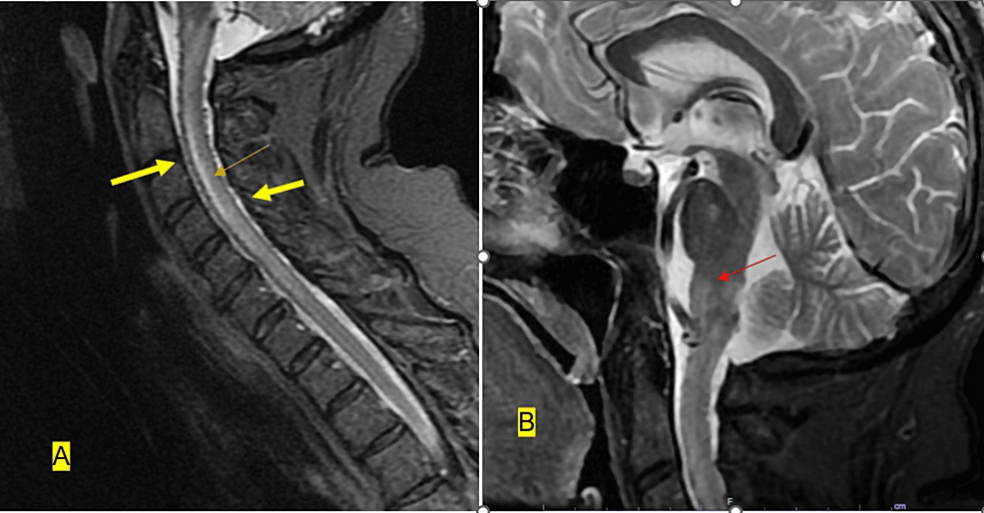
Cervical MRI with and without contrast Sagittal T2-weighted cervical MR demonstrating flow voids along (A) the ventral and dorsal surface of the medulla and cervical spinal cord (yellow arrows), linear cervical cord edema (orange arrow), and (B) edema within the pons and medulla (red arrow). As suspected on the prior MRI of the brain, there was a high T2 signal in the lower medulla and spinal cord, extending all the way down to the bottom of C6. It involved the posterior two-thirds of the cord most of the way, although, at the level of C2, it involved nearly the entire cross-section of the cord. On the sagittal T2 and STIR images, there was prominence of the posterior and anterior spinal arteries, which had a somewhat sawtooth appearance. Enhancement: There was a patchy enhancement in the cervical medullary junction and upper cord at the level of C1 seen on axial series 3 images 2 through 5 and post-contrast sagittal T1 image 7.
